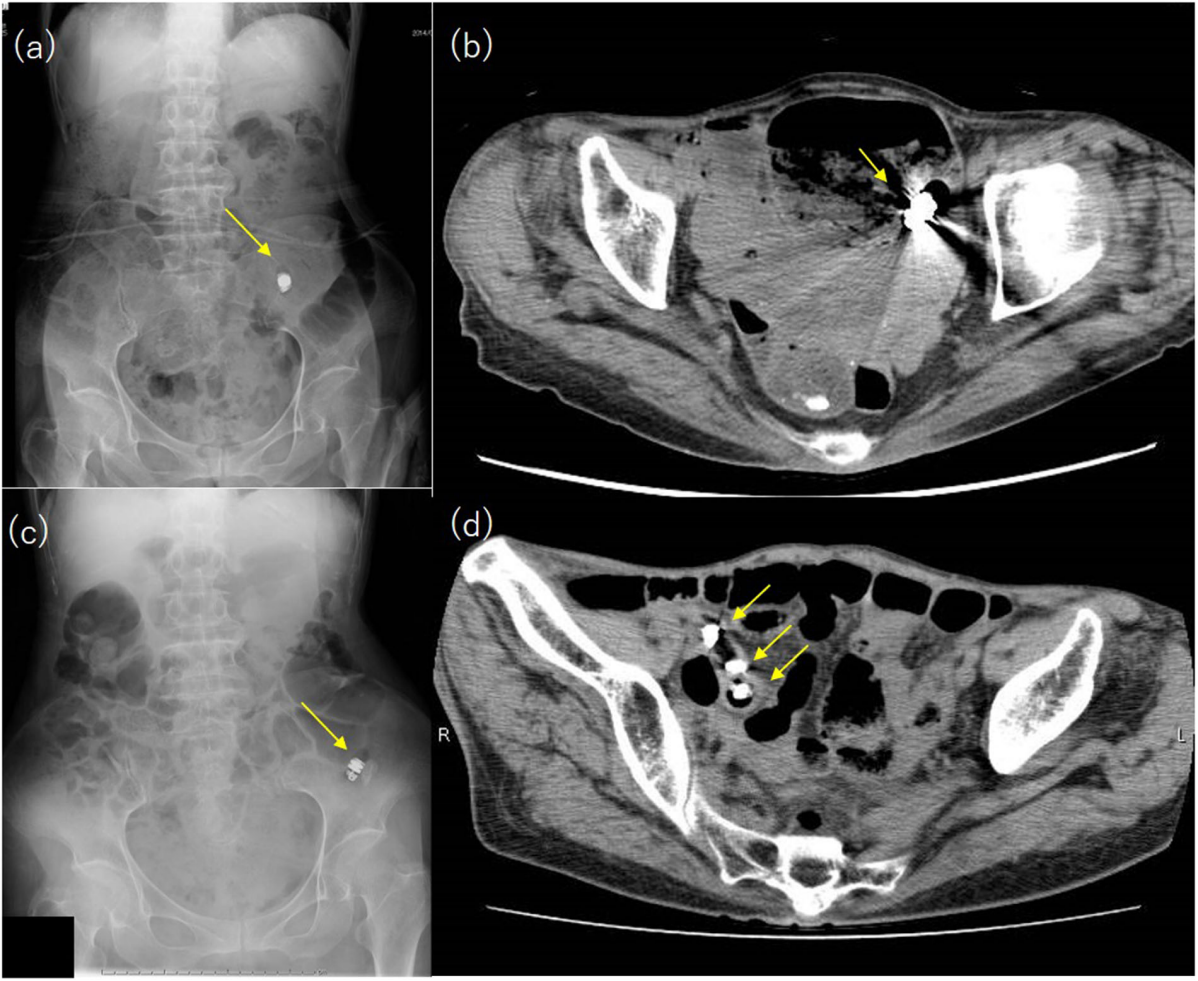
Radiography (a) and computed tomography (CT) (b) images taken one week after capsule endoscopy revealed retention of a capsule. Radiography images after 18 months revealed the existence of a capsule, which was not disrupted yet (c). CT image taken 20 months after capsule endoscopy revealed a fragmented capsule. The capsule was divided into three components (d) (arrows)
